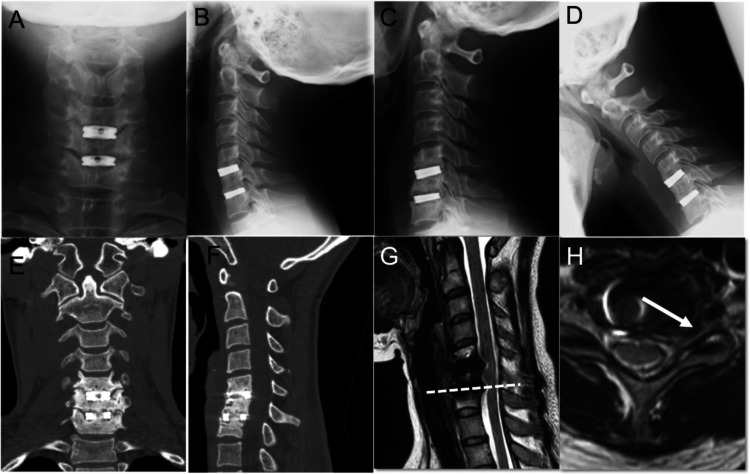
Preoperative radiological findings of Case 2 Titanium spacers were observed at the C5/C6 and C6/C7 levels. Cervical instability was not observed (A: anteroposterior X-ray image, B-D: lateral X-ray images). Calcification was observed in the titanium cages. Complete bony fusion was not achieved on computed tomography (E: axial image, F: sagittal image). The left nerve root of C7 was compressed owing to the presence of osteophytes (G and H: magnetic resonance images).
